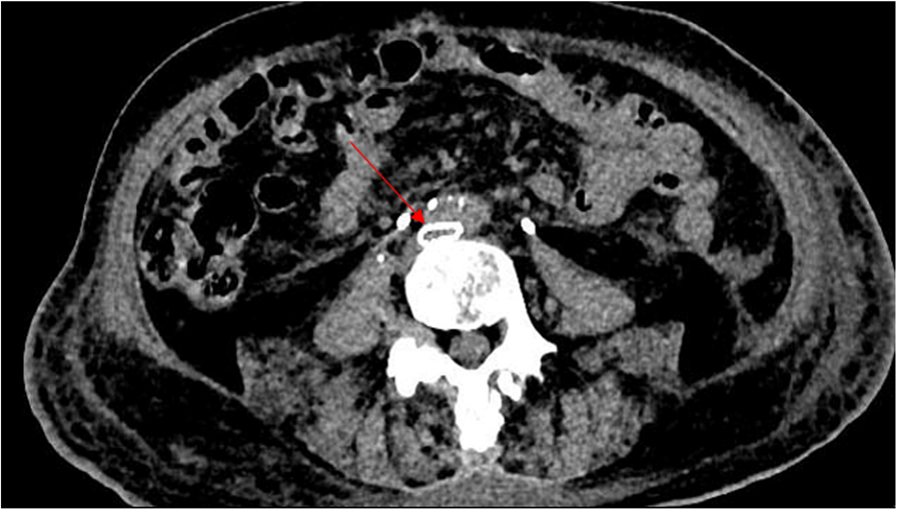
A patient was performed stent implantation in left iliac vein one year ago and recent CT result showed that the left iliac vein was compressed again
